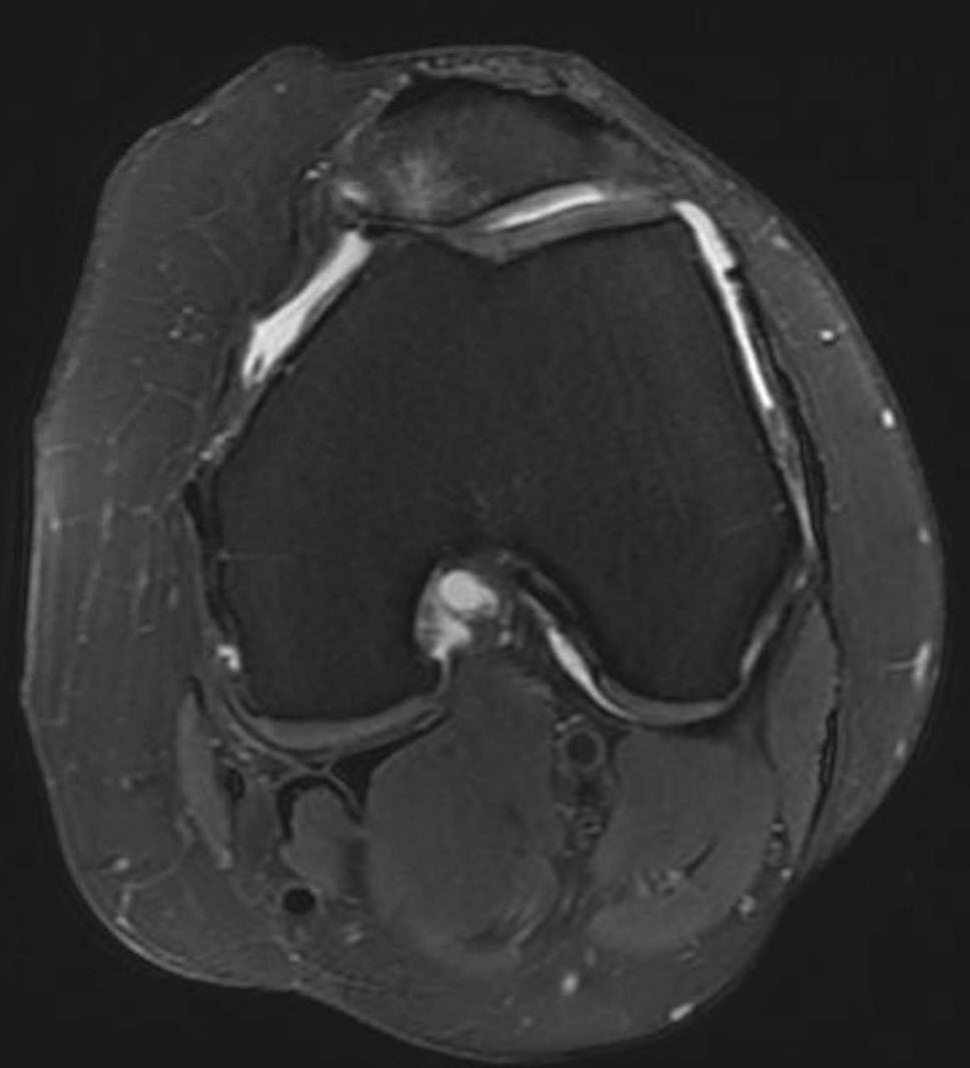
Transverse MRI slice showing medialized positioning of the patella with consecutive medial patellofemoral cartilage damage and bone bruise at the medial patellar facet
